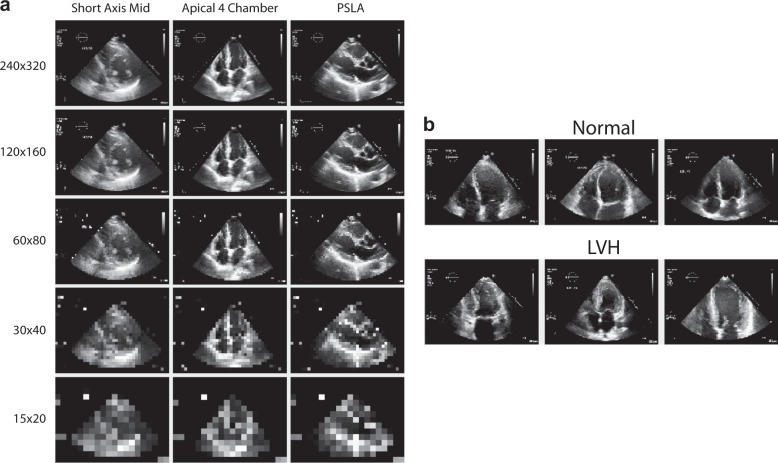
Sample data from echocardiographic studies. a Sample echocardiogram images at varying resolutions (rows) for three example views (columns). Selection of the optimal resolution is influenced by the tradeoff between classifier performance and computational time. b Sample apical four chamber echocardiography images with and without Left Ventricular Hypertrophy (LVH). LVH is characterized by the thickening of the left ventricle—a perilous condition increasing the risk of myocardial infarction, stroke, and death
